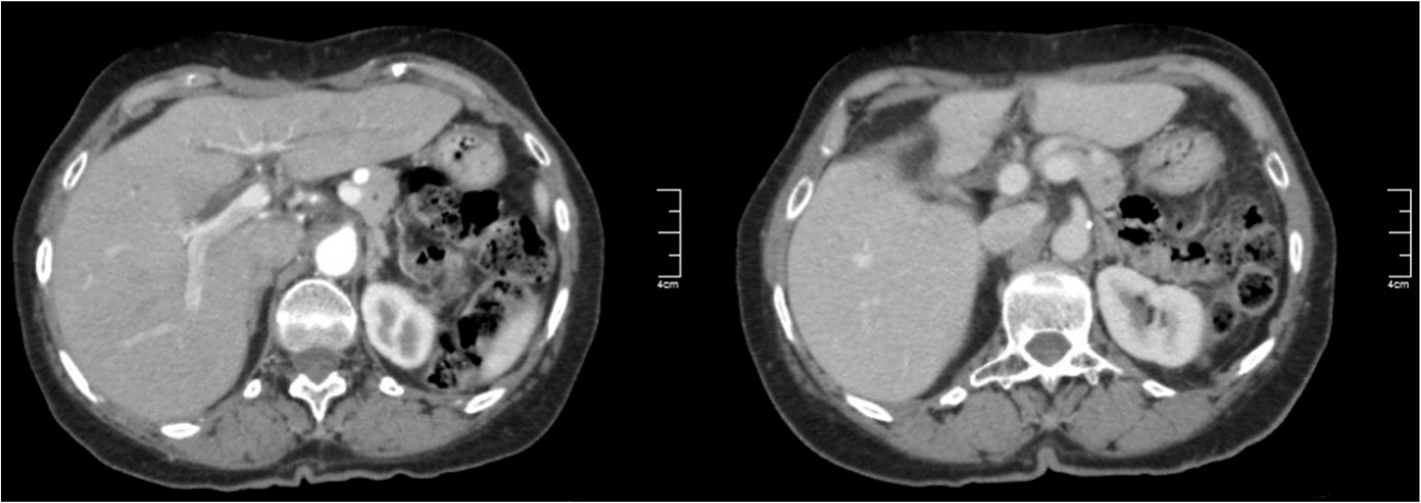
Postoperative CT scans: transverse section imaging showing no signs of recurrence 8 months after surgery
